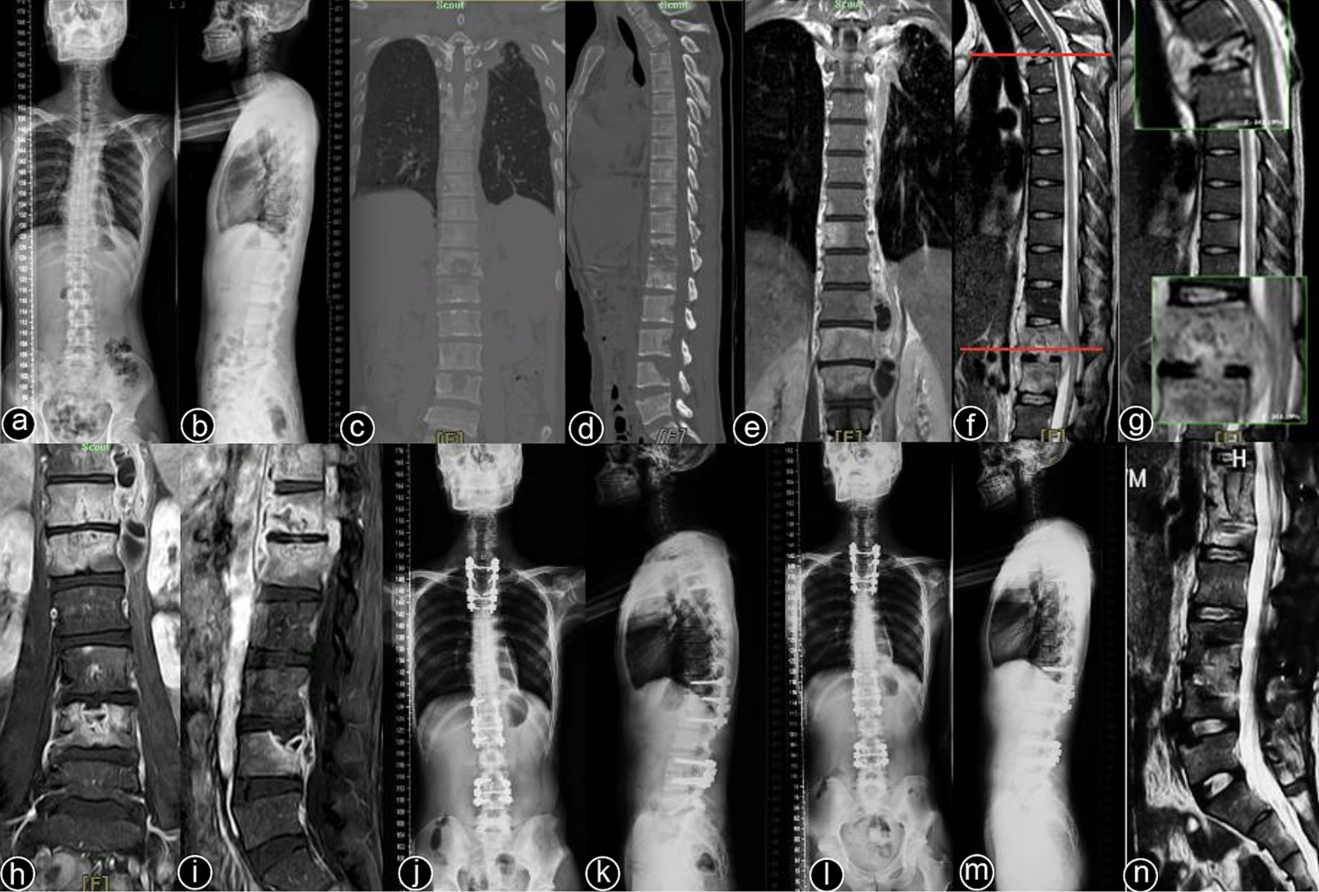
Patient, male, 48 years old. Diagnosis: spinal tuberculosis (T3, T12–L1, L3–L4). Surgical approach: non-intervertebral surgery at T1–T4 and T11–L1; intervertebral surgery at L3–L4. (A,B) Preoperative full-spine anteroposterior and lateral X-rays show unclear visualization of the T3 vertebral body, with roughened anterior margins and narrowed intervertebral spaces at T12–L1 and L3–L4. (C,D) Preoperative full-spine CT reveals multiple non-contiguous vertebral body destructions with paravertebral soft tissue swelling. (E–I) Preoperative thoracic and lumbar MRI show abnormal signal intensities in multiple thoracic and lumbar vertebrae, with narrowed intervertebral spaces and extensive paravertebral abscess formation. (J,K) Immediate postoperative full-spine anteroposterior and lateral X-rays show stable internal fixation at T1–T4, T12–L1, and L3–4. (L–N) One-year postoperative full-spine anteroposterior and lateral X-rays, and lumbar MRI show no loosening or breakage of the internal fixation, a clear spinal canal, and no recurrence of the local lesion.
